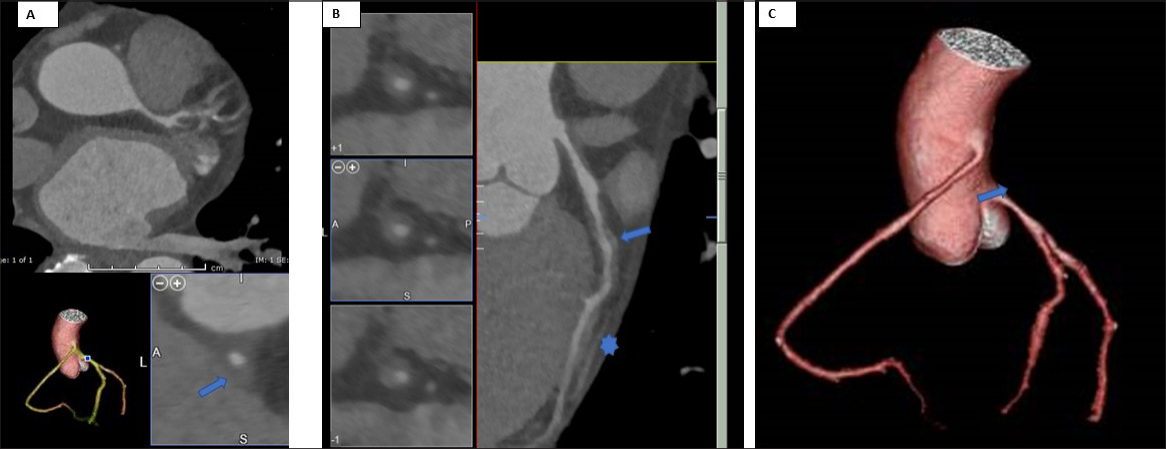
Coronary computed tomographic angiography (CCTA). (A) Diffuse narrowing of the left main artery with 70-80% maximal stenosis; CCTA reformat depicts diffuse narrowing of the left main artery resulting in 40-50% stenosis (arrow). (B) Curve planar reformat of the left anterior descending (LAD) shows diffuse circumferential wall thickening of the proximal LAD resulting in up to 50% stenosis (arrow). Incidental 1.4-cm myocardial bridge involves the mid-LAD without extrinsic compression or significant intraluminal stenosis (*). (C) 3-dimensional volume rendering of the coronary tree demonstrates high takeoff of the right coronary artery from the anterior surface of the ascending aorta (arrow).
